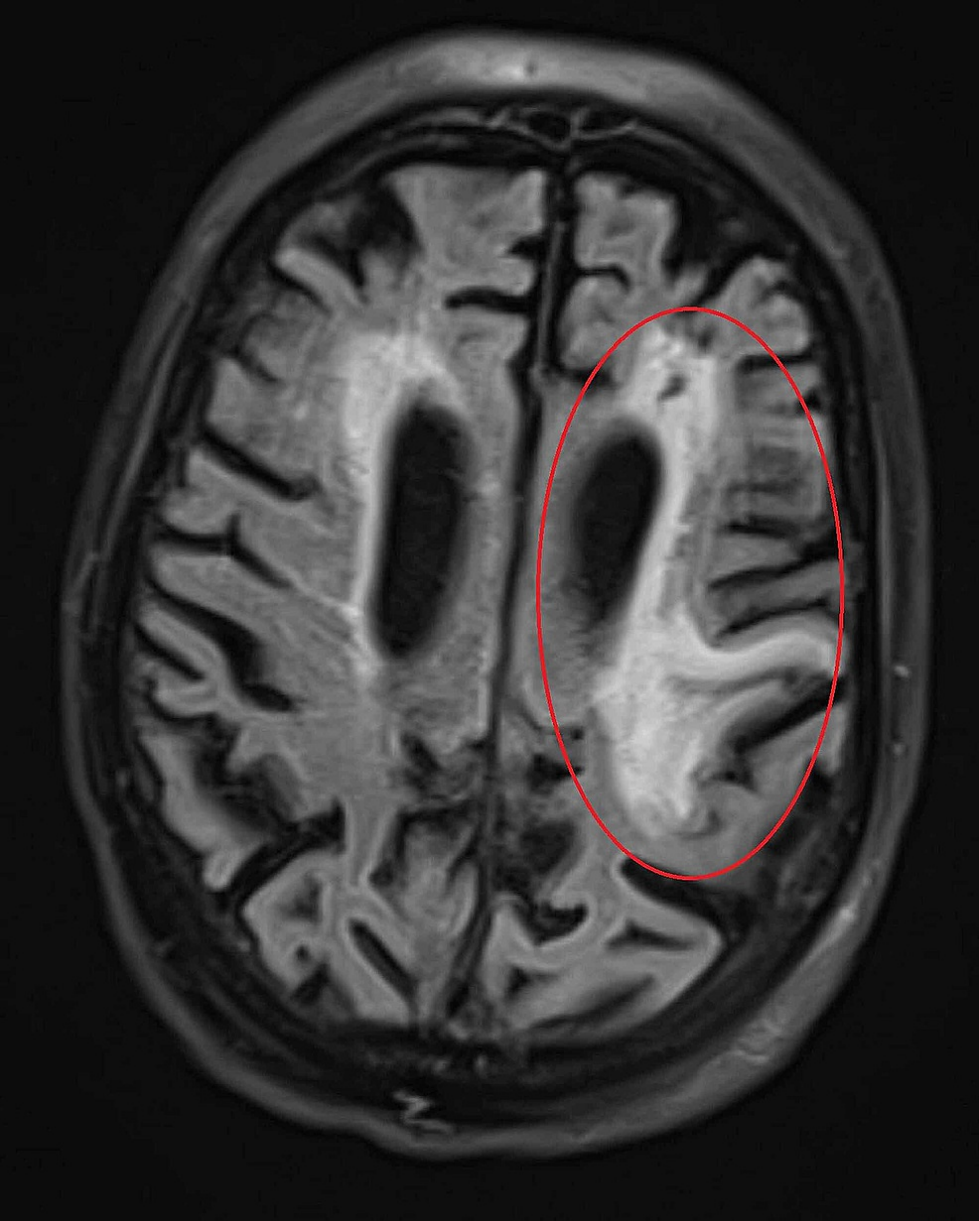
MR axial FLAIR sequence showing increased signal in left frontal lobe representing chronic encephalomalacia. MR = magnetic resonance FLAIR = fluid-attenuated inversion recovery
